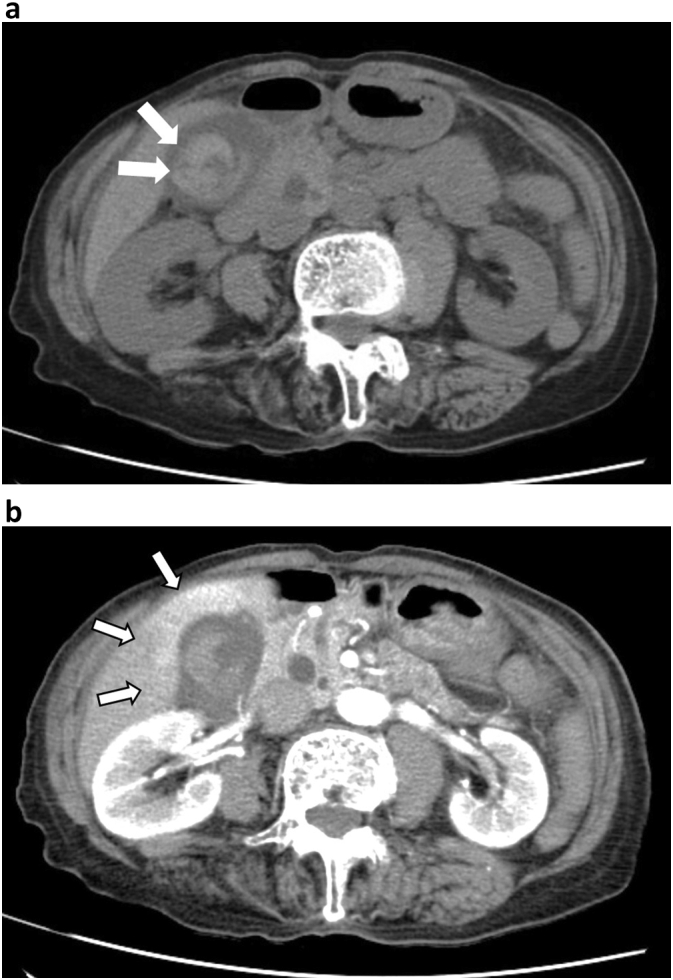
a. Findings on computed tomography. Computed tomography showed a tense gallbladder with a heterogeneous, high-density area (arrows). b. Findings on contrast-enhanced computed tomography in the arterial phase. Contrast-enhanced computed tomography showed increased pericholecystic attenuation in the arterial phase (arrows), which suggests acute cholecystitis.
